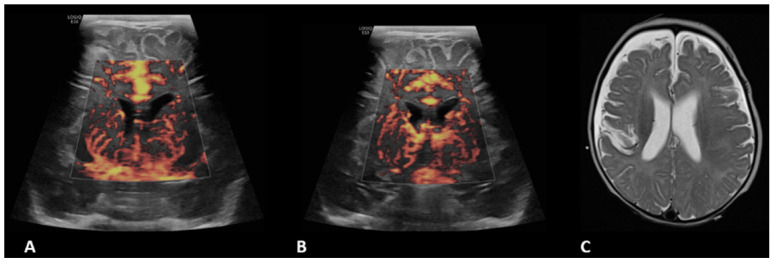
A 4-month-old boy with a history of complete common atrioventricular canal underwent ECMO due to failure to wean from cardiovascular bypass after major cardiac surgery. Brain MVI was performed after five days from cannulation; grayscale US (not shown) demonstrated extra-axial collections. Coronal brain MVI (A,B) demonstrated marked cortical white matter vascular engorgement and heterogeneity. MRI obtained three months after ECMO decannulation showed a moderate, late subacute to chronic infarct in the right parietotemporal cortex (C).
